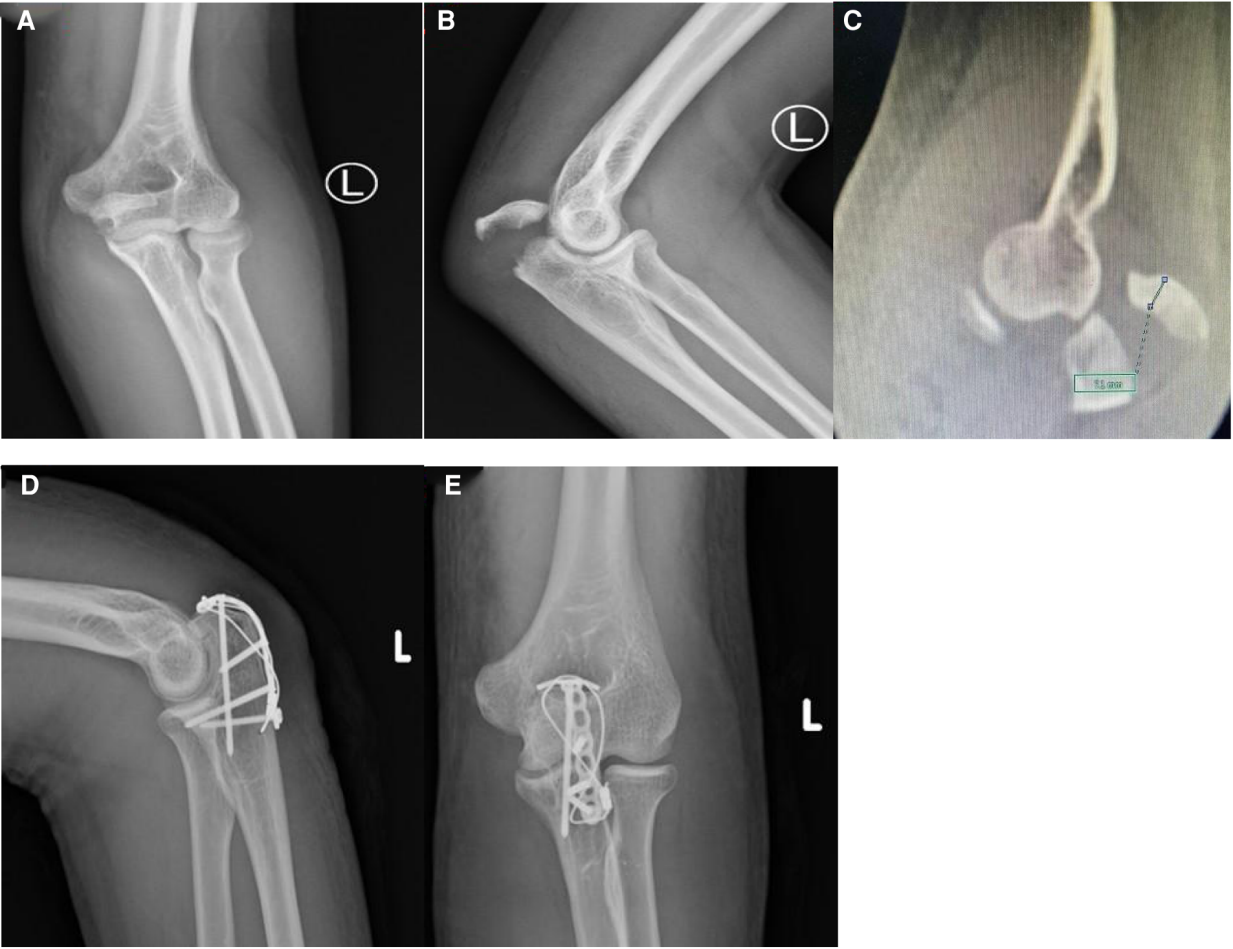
A 30-year-old male with olecranon avulsion caused by a traffic accident, treated by a T-plate combined with wire fixation; final elbow functional results were good; x-ray and CT of the elbow before surgery (A–C); x-ray of elbow after surgery (D,E).
